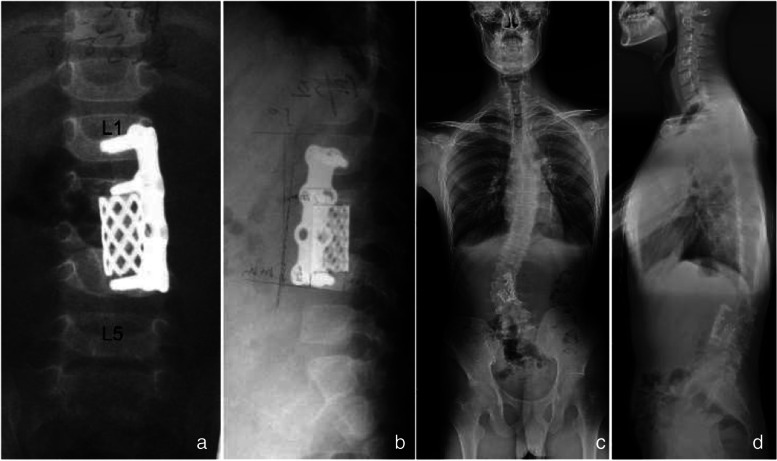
A 3-year-old boy with L2-L4 tuberculosis underwent anterior debridement and reconstruction using a titanium mesh and plate. Three-month postoperative X-ray showed good lumbar spine alignment and internal fixation position (a, b). Spinal deformity was observed at 15 years postoperatively (c, d)
